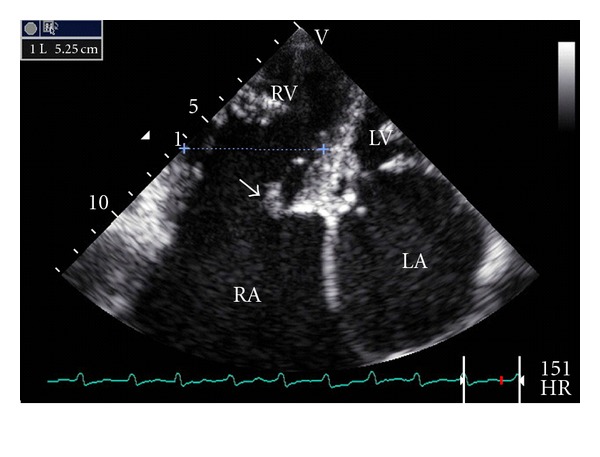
Transthoracic echocardiography, four apical four chambers view. Dilatation of the right ventricle (52.5 mm, mild diameter). The tricuspid valve was thickened and not displaced (white arrow).
